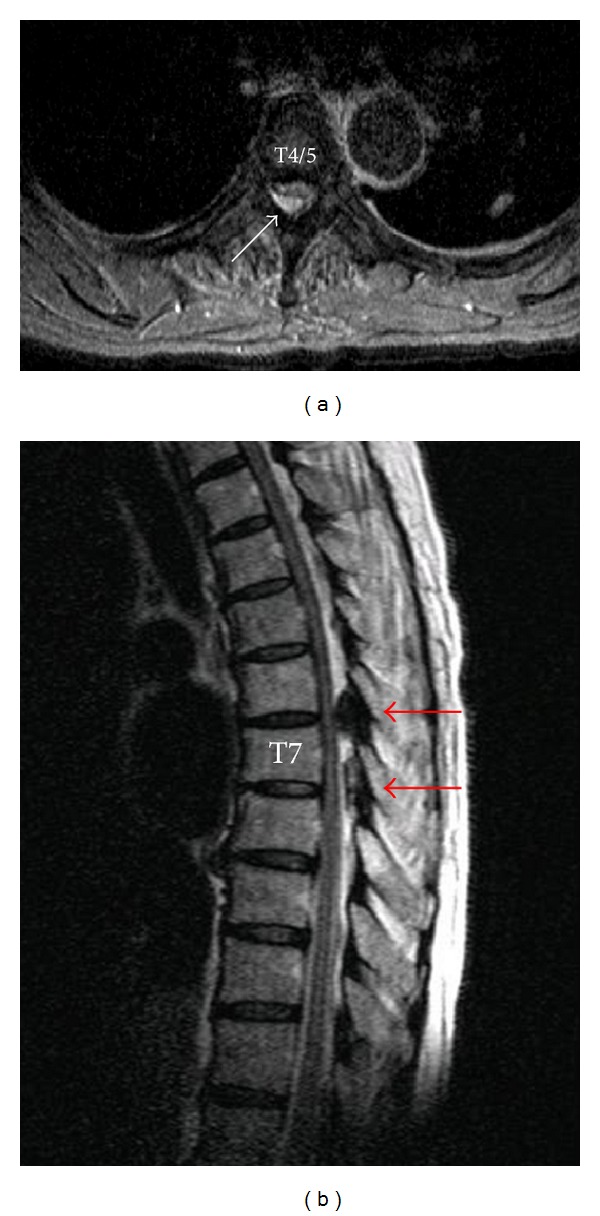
Magnetic resonance images one hour after onset of paraplegia. (a) T2-weighted image. Arrow indicates hematoma compressing the dural sac at T4/5. (b) T2-weighted image. Arrows indicate ossification of the posterior longitudinal ligament at T6/7 and T7/8.
